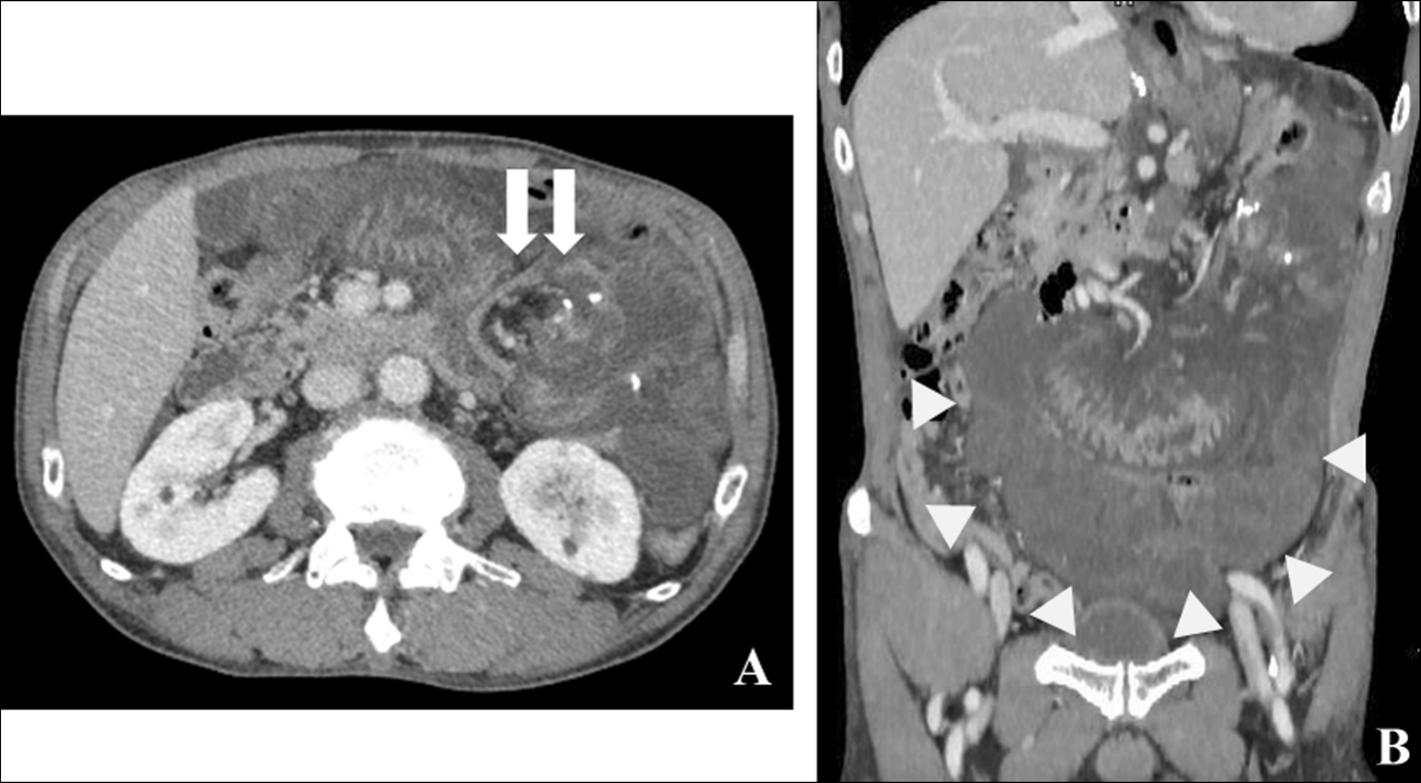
Abdominal CT scan showing whirl sign (*white arrows*), ascites, and closed-loop formation of the small intestine (*white arrowheads*): a axial section; b coronal section
